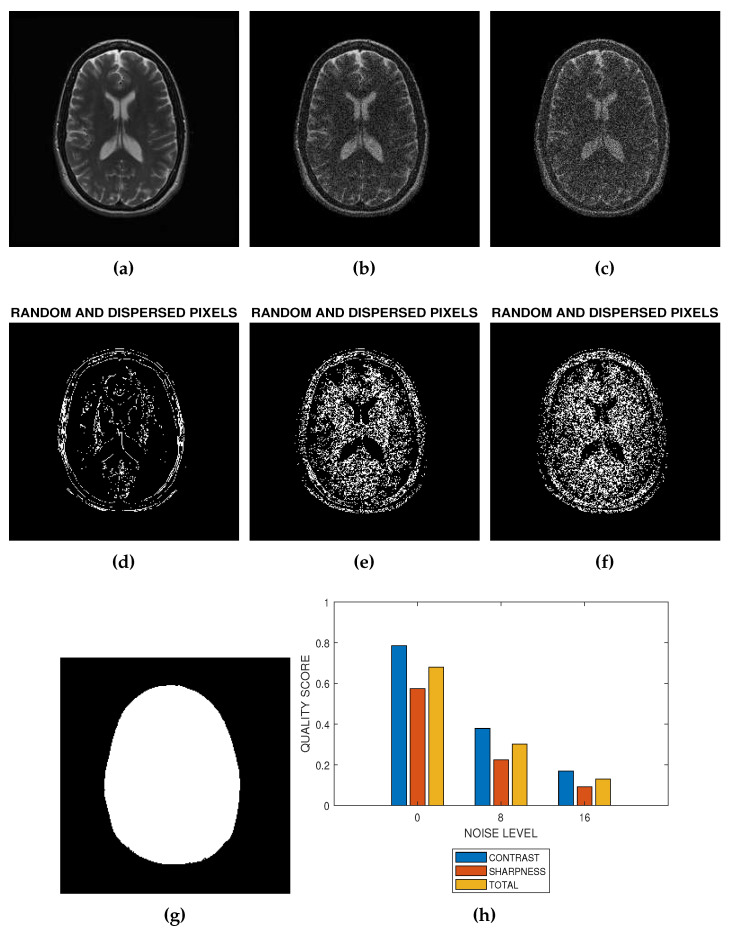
The implementation of blind noise-based quality assessment in MRI images. (a) The test image provided by NeuroRx Research Inc. (b) Degraded version of the test image in (a) at 8 percent Rician noise. (c) Degraded version of the test image in (a) at 16 percent Rician noise. (d) Random and dispersed pixels in the test image in the absence of noise distortion. (e) Random and dispersed pixels in the test image at 8 percent Rician noise. (f) Random and dispersed pixels in the test image at 16 percent Rician noise. (g) The Foreground extracted from the test image in (a). (h) Variation of contrast, sharpness and total quality scores at Rician noise levels of 0 percent, 8 percent and 16 percent.
